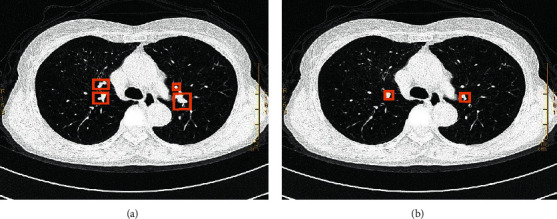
Changes in lung cancer CT images before and after chemotherapy. Male, 79 years old, patient with lung cancer. (a) Before chemotherapy, the CT image showed the distribution of nodular soft tissue density masses at the posterior and lower right hilum (the size was about 0.8 cm in diameter, the edges were slightly under-rectified, and there was no obvious swollen lymphoma in the mediastinum). (b) After chemotherapy, CT images showed that the posterior and lower right hilum nodules were significantly smaller than before treatment, with a diameter of about 0.4 cm, blurred edges, reduced right lung volume, and no obvious lymphadenopathy in the mediastinum.
